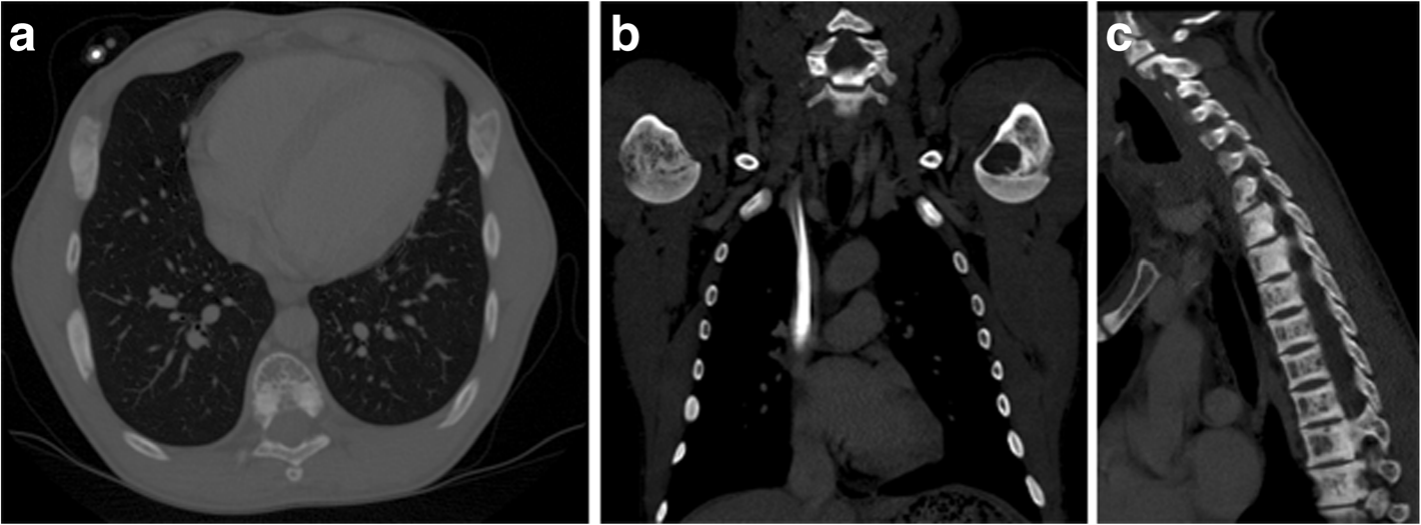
a–c Patients with secondary hyperparathyroidism due to chronic renal failure. CT demonstrated asymptomatic bilateral mixed lytic/sclerotic expansile rib lesions, lytic lesion in the left humeral head and the rugger-jersey spine
